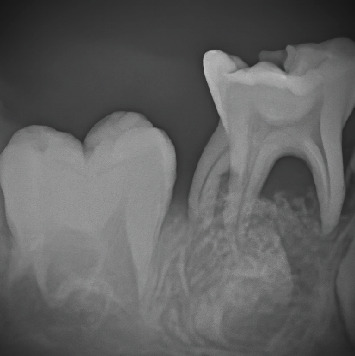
Periapical radiograph of Molar #85, where radiolucency in the furcation and an extremely deep carious lesion is observed.
